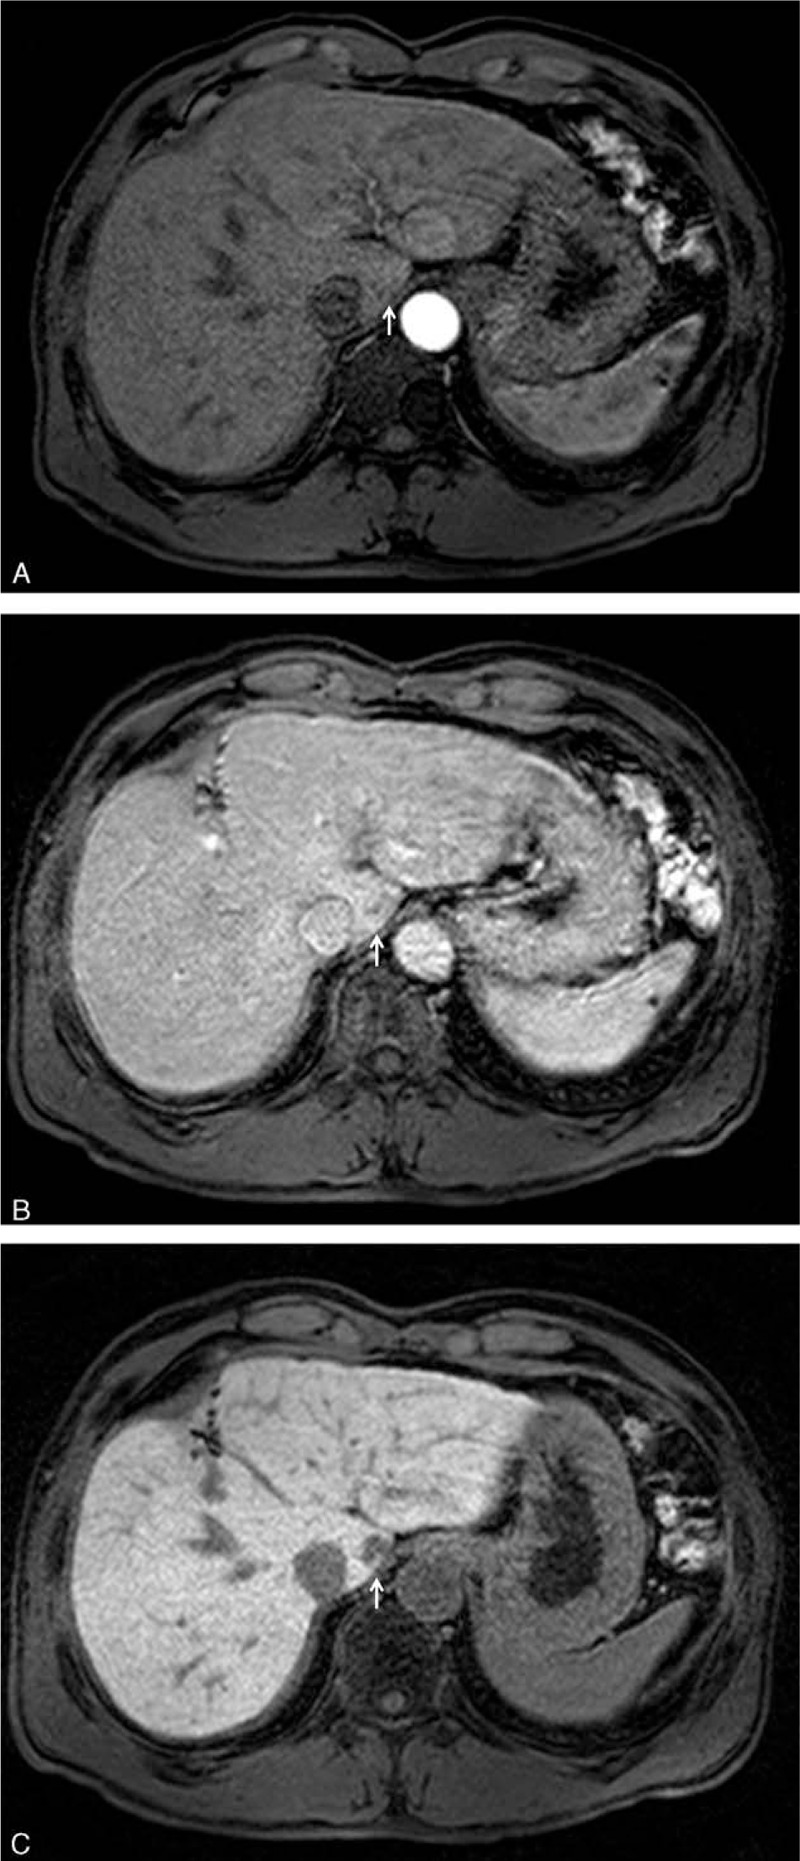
A 50-year-old man developed a 1.5-cm recurrent hepatocellular carcinoma 1 year after segmentectomy, which was detected with gadoxetic acid-enhanced magnetic resonance imaging but no lesion was found on the 3 months prior multidetector computed tomography (not shown). (A) Tumor enhanced on the arterial phase in segment 1 of the liver, appearing hyperintense to the background liver. (B) On the portal venous phase, tumor appeared hypointense to the background liver. (C) Tumor demonstrated no gadolinium ethoxybenzyl diethylentriamine pentaacetic acid uptake on the hepatobiliary phase.
